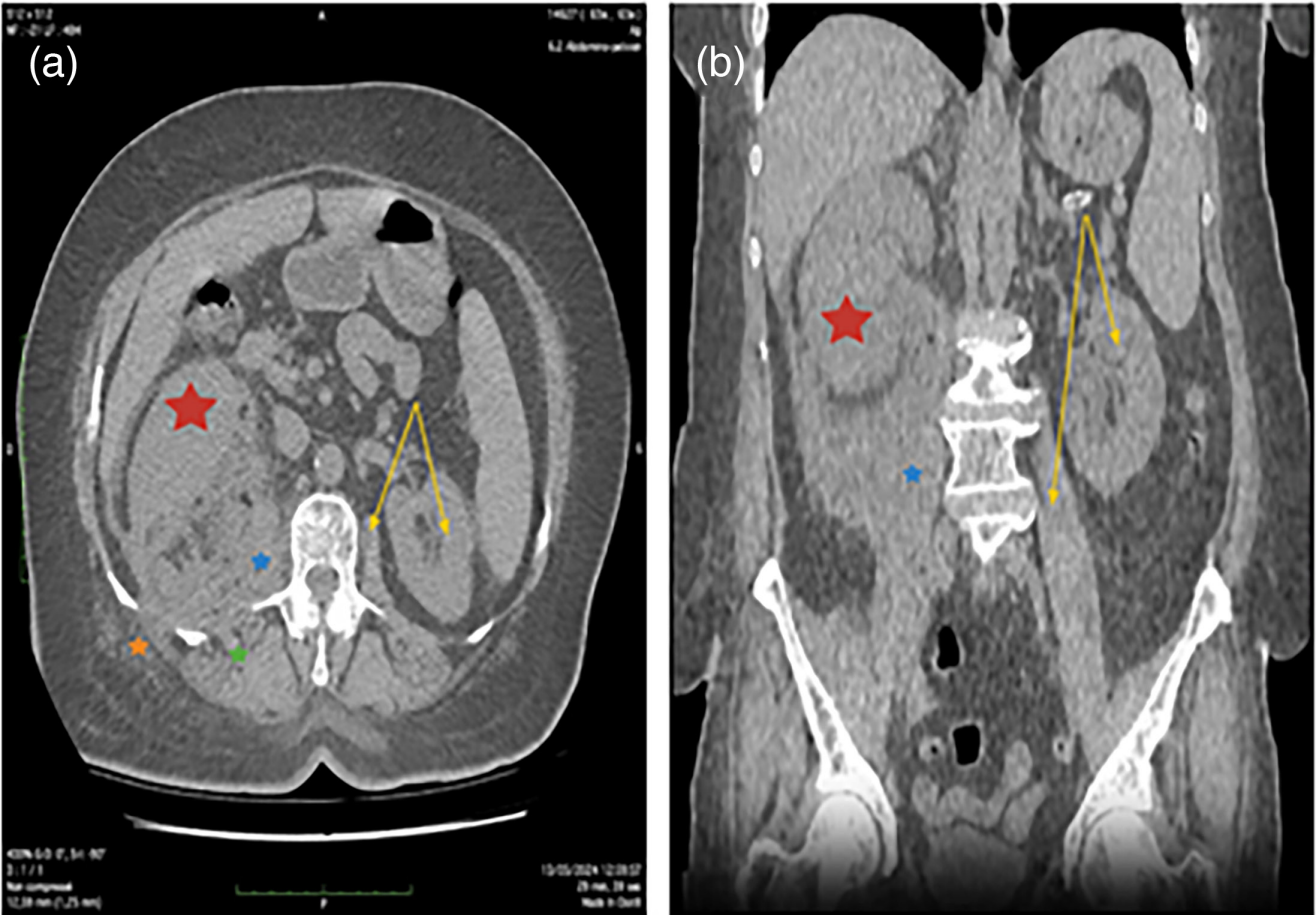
Axial (a) and coronal (b) views of a CT scan showing a destroyed right kidney (red star) with disappearance of the pyelocaliceal cavities (pyonephrosis) and perirenal phlegmon extending to the psoas muscle (blue star), the paravertebral muscles (green star) and the muscles of the posterior abdominal wall through the intercostal spaces (orange star). For comparison, the yellow arrow represents the non-infiltrated contralateral left side (kidney and psoas muscle).
